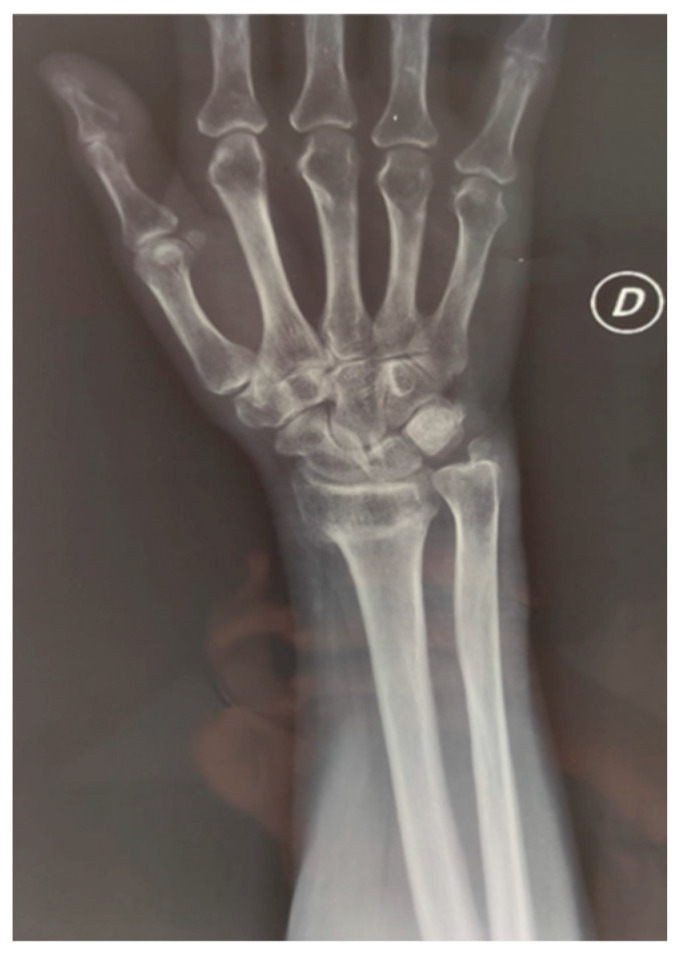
A classic finding of a distal radius fracture depicted by X-ray imaging.
